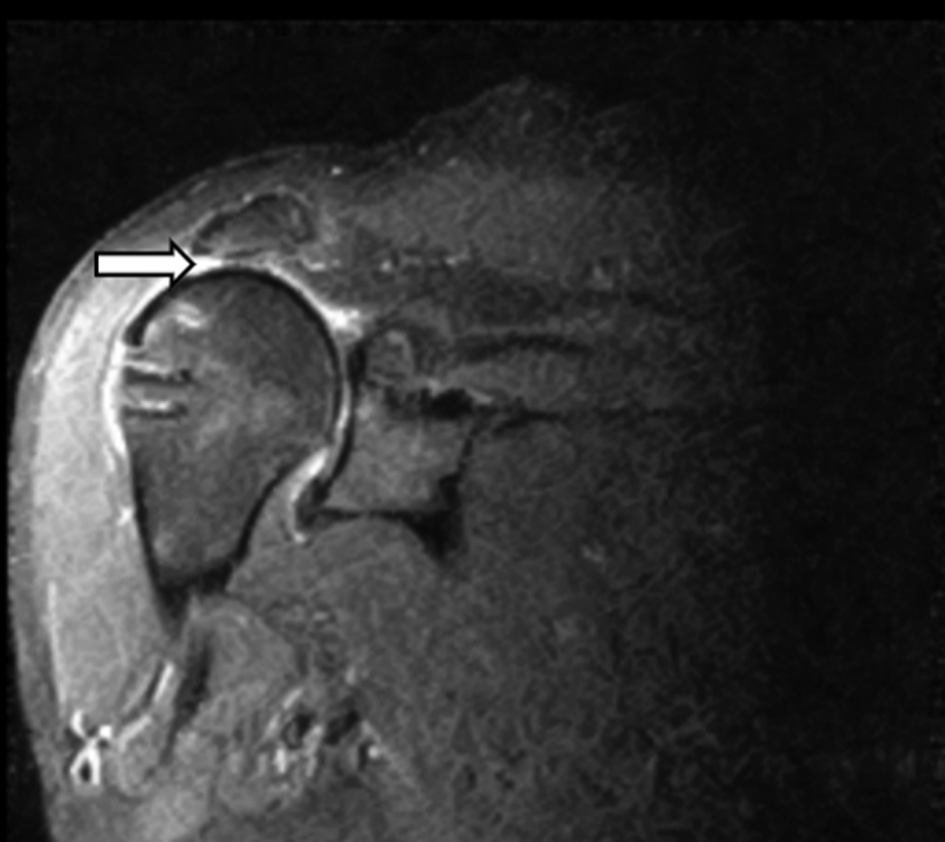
T2 inversion recovery coronal MRI image of the right shoulder. The image displays a postoperative MRI of a patient after superior capsular reconstruction with complete failure. The arrow denotes absence of graft between humerus and acromion. There is no attachment noted to the glenoid on the image which confirms a completely disrupted graft. Note humerus is nearly articulating with undersurface of acromion. (MRI, magnetic resonance imaging.)
